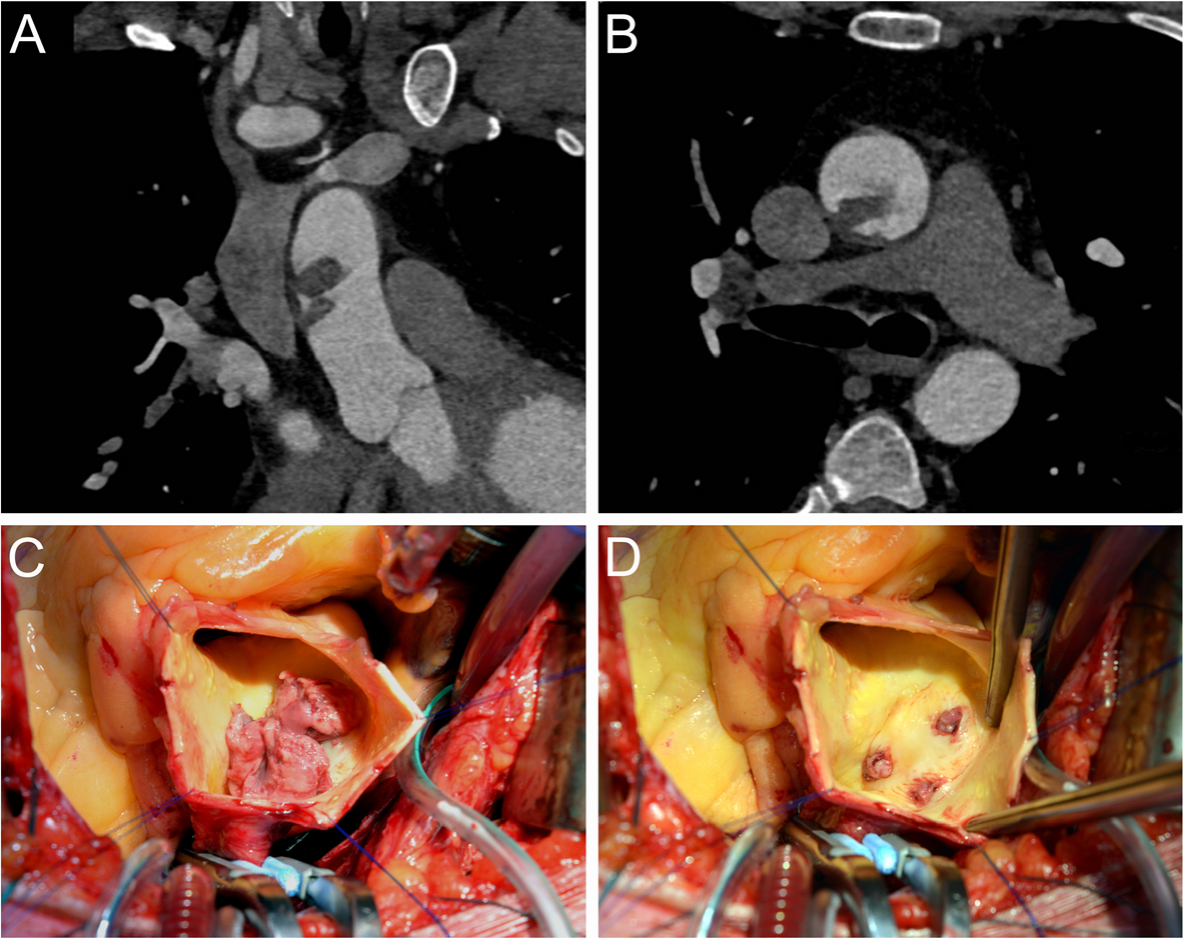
a-b Pre-operative computed tomography of the aorta showed a large structure in the ascending aorta. c-d Intra operative images of the large thrombus in the ascending aorta and the atherosclerotic insertion in the aortic wall
